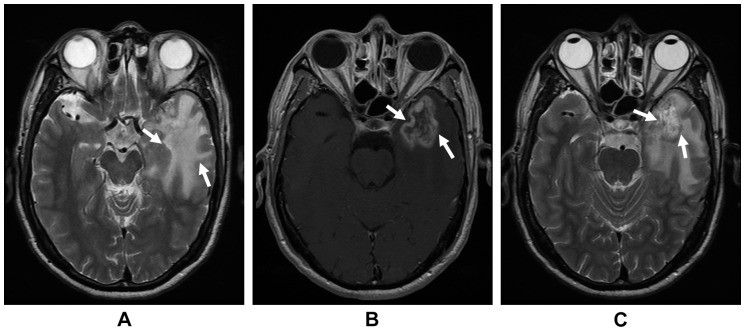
Representative MRI of patients with radiation-induced temporal lobe necrosis. (a) the T2-weighted axial image shows a finger-like lesion of increased signal intensity (between the arrows); (b) the post-contrast T1-weighted axial image shows a lesion with necrosis and heterogeneous signal abnormalities (between the arrows); (c) the T2-weighted axial image shows an oval cyst with high T2 signal intensity (between the arrows).
